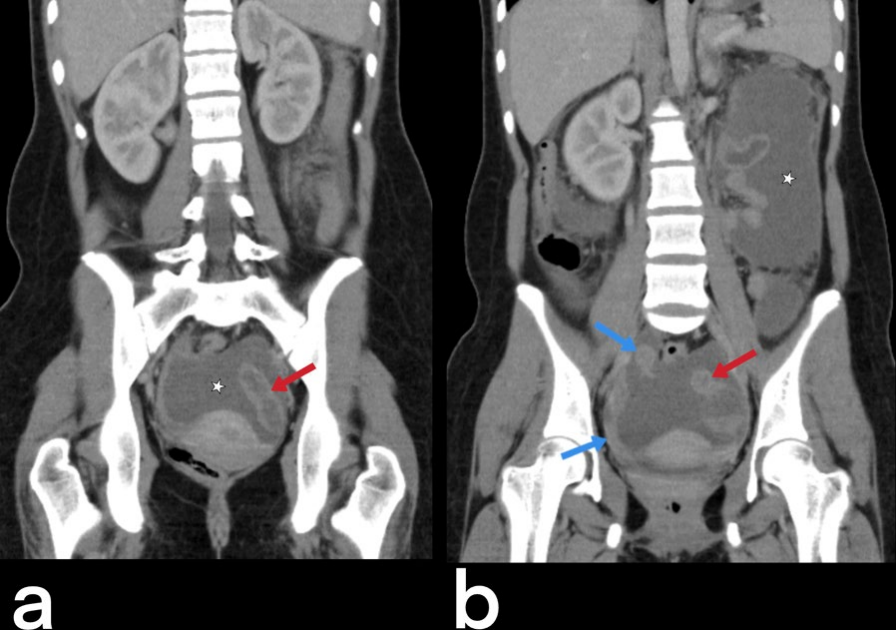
Bilaterally hydrosalpinx (right: blue arrows, left: red arrow) and ascites (stars) in the peritoneal cavity are observed on coronal contrast-enhancement CT images (a, b) of the same patient (in Fig. 41)
